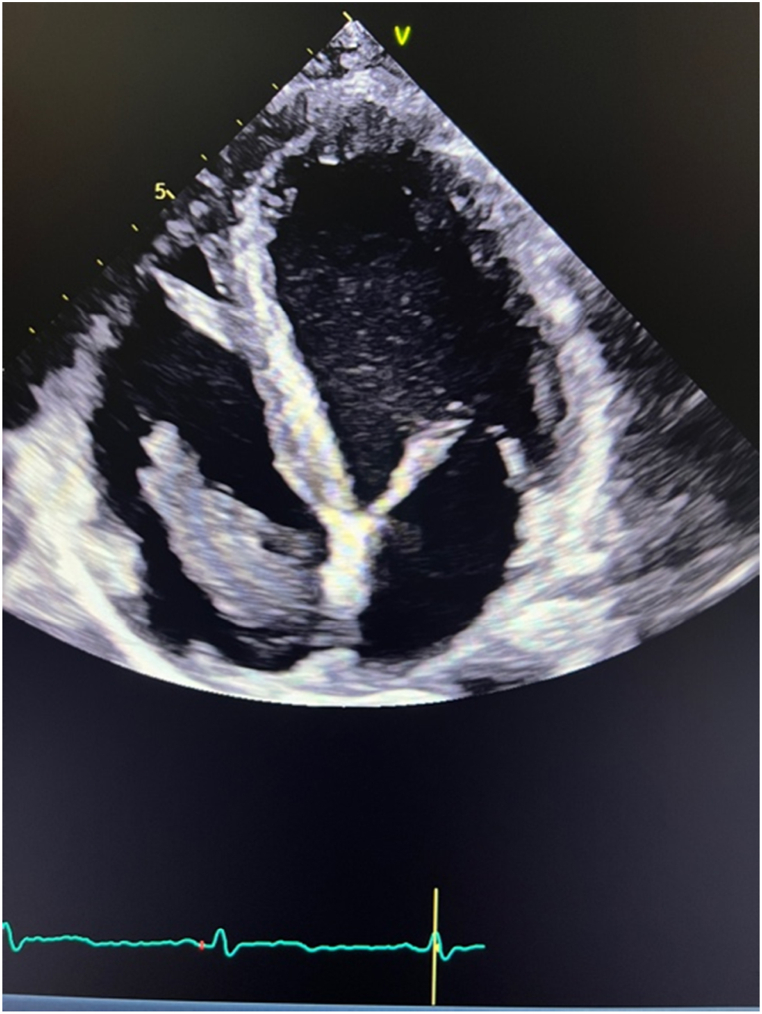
Tumoral thrombosis in right atrium and ventricle with a normal left and right ventricular function on echocardiography.
